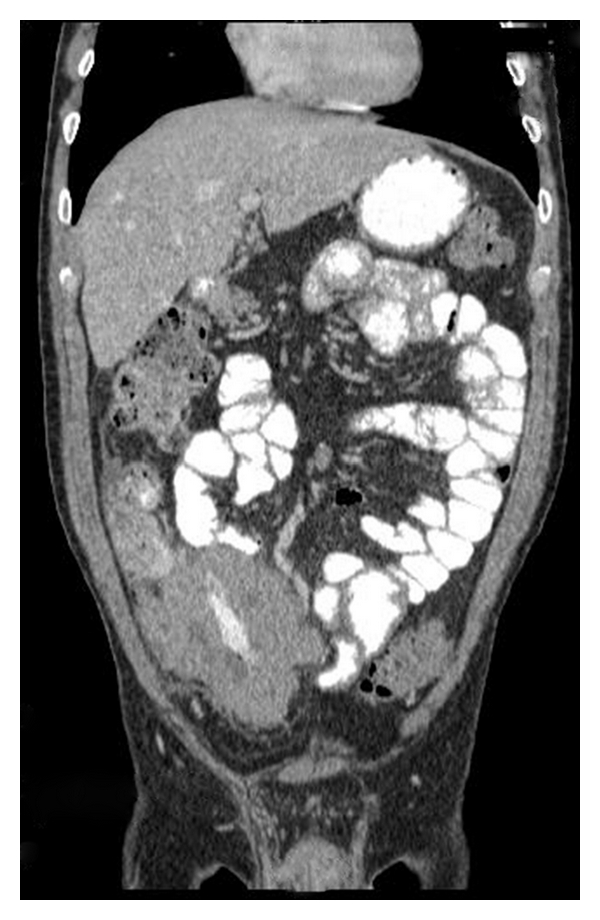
Coronal reformatted multislice abdominal computed tomography displaying a terminal ileum segment in the right-lower quadrant with marked thickening when compared to the adjacent ileal segments and showing mild enhancement after oral and intravenous contrast agent administration.
